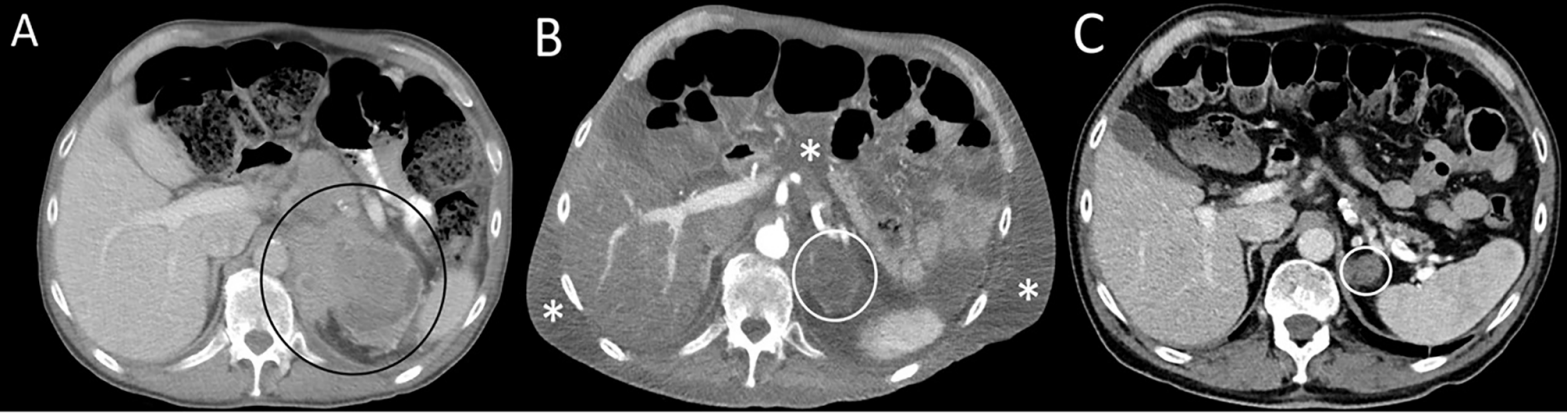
Serial axial computed contrast-enhanced computed tomography (CT) images of the upper abdomen demonstrate (A) a left adrenal mass (circle) 4 months before admission and prior to treatment, (B) decreased tumor size by more than 50% (circle) as well as new-onset ascites and soft tissue edema (asterisks) at the time of hospital admission following therapy with combination immune checkpoint inhibitor therapy; (C) resolution of ascites and soft tissue edema and continued tumor response (circle) 8 months after admission. The patient was not receiving therapy at the time of the follow-up CT.
